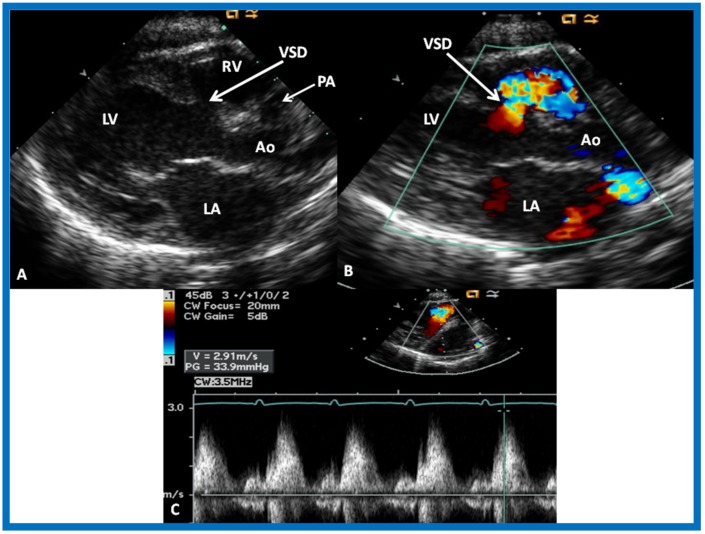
Selected video frames from precordial long axis views of a neonate with tricuspid atresia with normally related great arteries demonstrating enlarged left atrium (LA) and left ventricle (LV), a small right ventricle (RV) and a moderate sized ventricular septal defect (VSD) (thick arrow) on 2D (A) and color flow (B) imaging. Turbulent flow (B) with a Doppler flow velocity of 2.91 m/s by continuous wave Doppler (C) suggests some restriction of the VSD. Ao, Aorta; PA, pulmonary artery. Reproduced from Rao P.S. [39].
